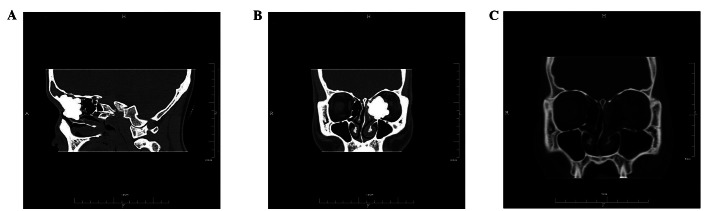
(A) An osseous lesion in the ethmoid sinus extending into the base of the frontal sinus. (B) An osseous lesion in the left ethmoid sinus extending into the orbit. (C) A computed tomography (CT) scan obtained 1 week after surgery revealed no evidence of the osteoma in coronal CT images.
